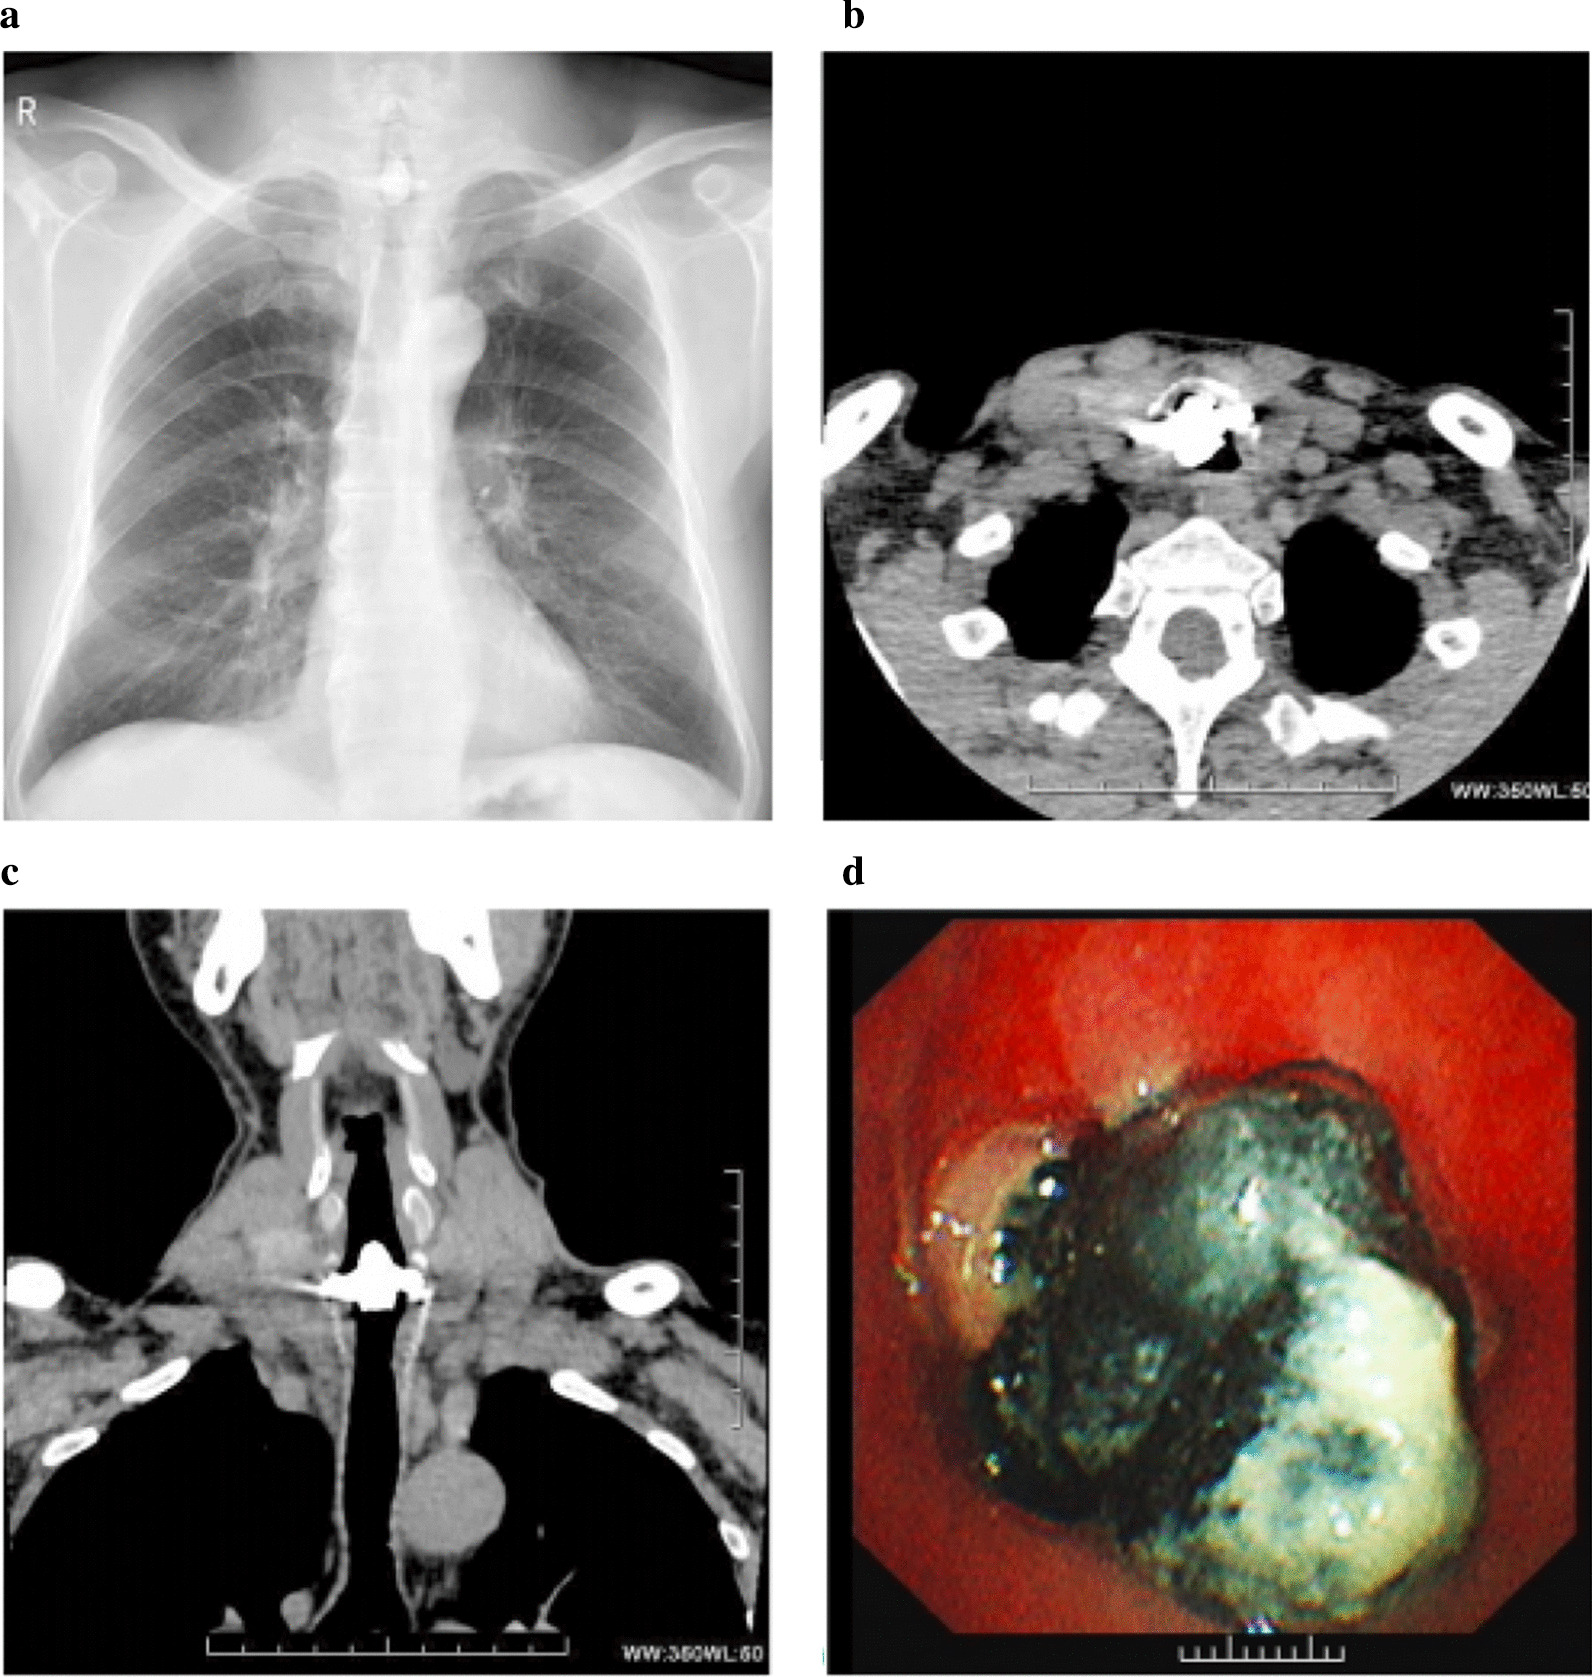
Radiological examinations and fiberoptic bronchoscopy were performed on a 49‑year‑old man with a tracheal coal foreign body prior to surgery. a Chest radiograph shows a high‑density nodule (arrows) in the trachea about the level of the 7th cervical vertebra. b, c Chest axial and coronal CT images also reveal this high‑density nodule (arrows) inserted from the trachea into the right thyroid at the same level as seen on the chest radiograph. d Fiberoptic bronchoscopy reveals a black foreign body in the subglottic trachea. The tracheal lumen was mostly blocked and the fiberoptic bronchoscopy failed to pass through the trachea due to its severe stenosis
